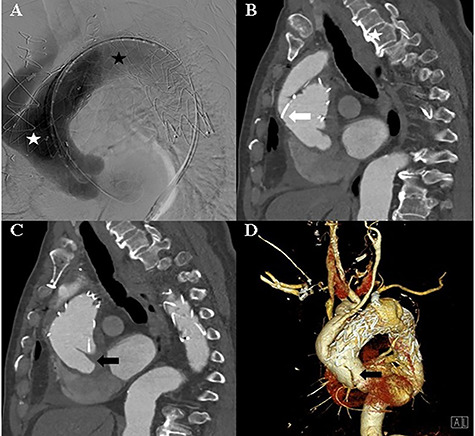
A: Aortic angiography during TEVAR shows the thoracic graft implanted in the ascending thoracic aorta and aortic arch (white asterisk) and the thoracic graft implanted in the aortic arch and descending thoracic aorta (black asterisk). B: Computed tomography of the post-TEVAR shows the placed thoracic graft (white arrow). C and D: Computed tomography of the post-TEVAR shows the intimal dissecting flap at the proximal ascending aorta.
